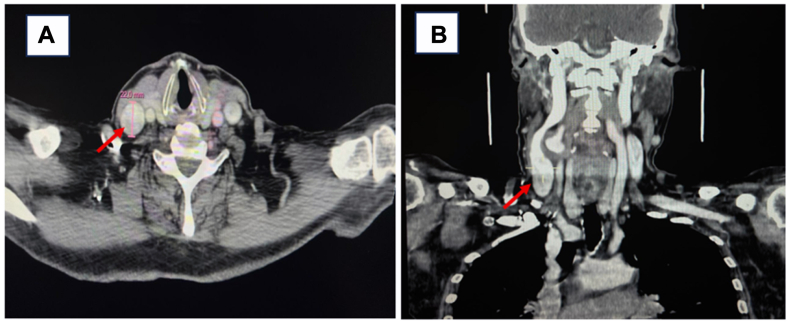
Cervical computed tomography angiography showing a saccular aneurysm of the right common carotid artery, measuring 22 mm, visible on axial (A) and coronal (B) views (*red arrows*).
